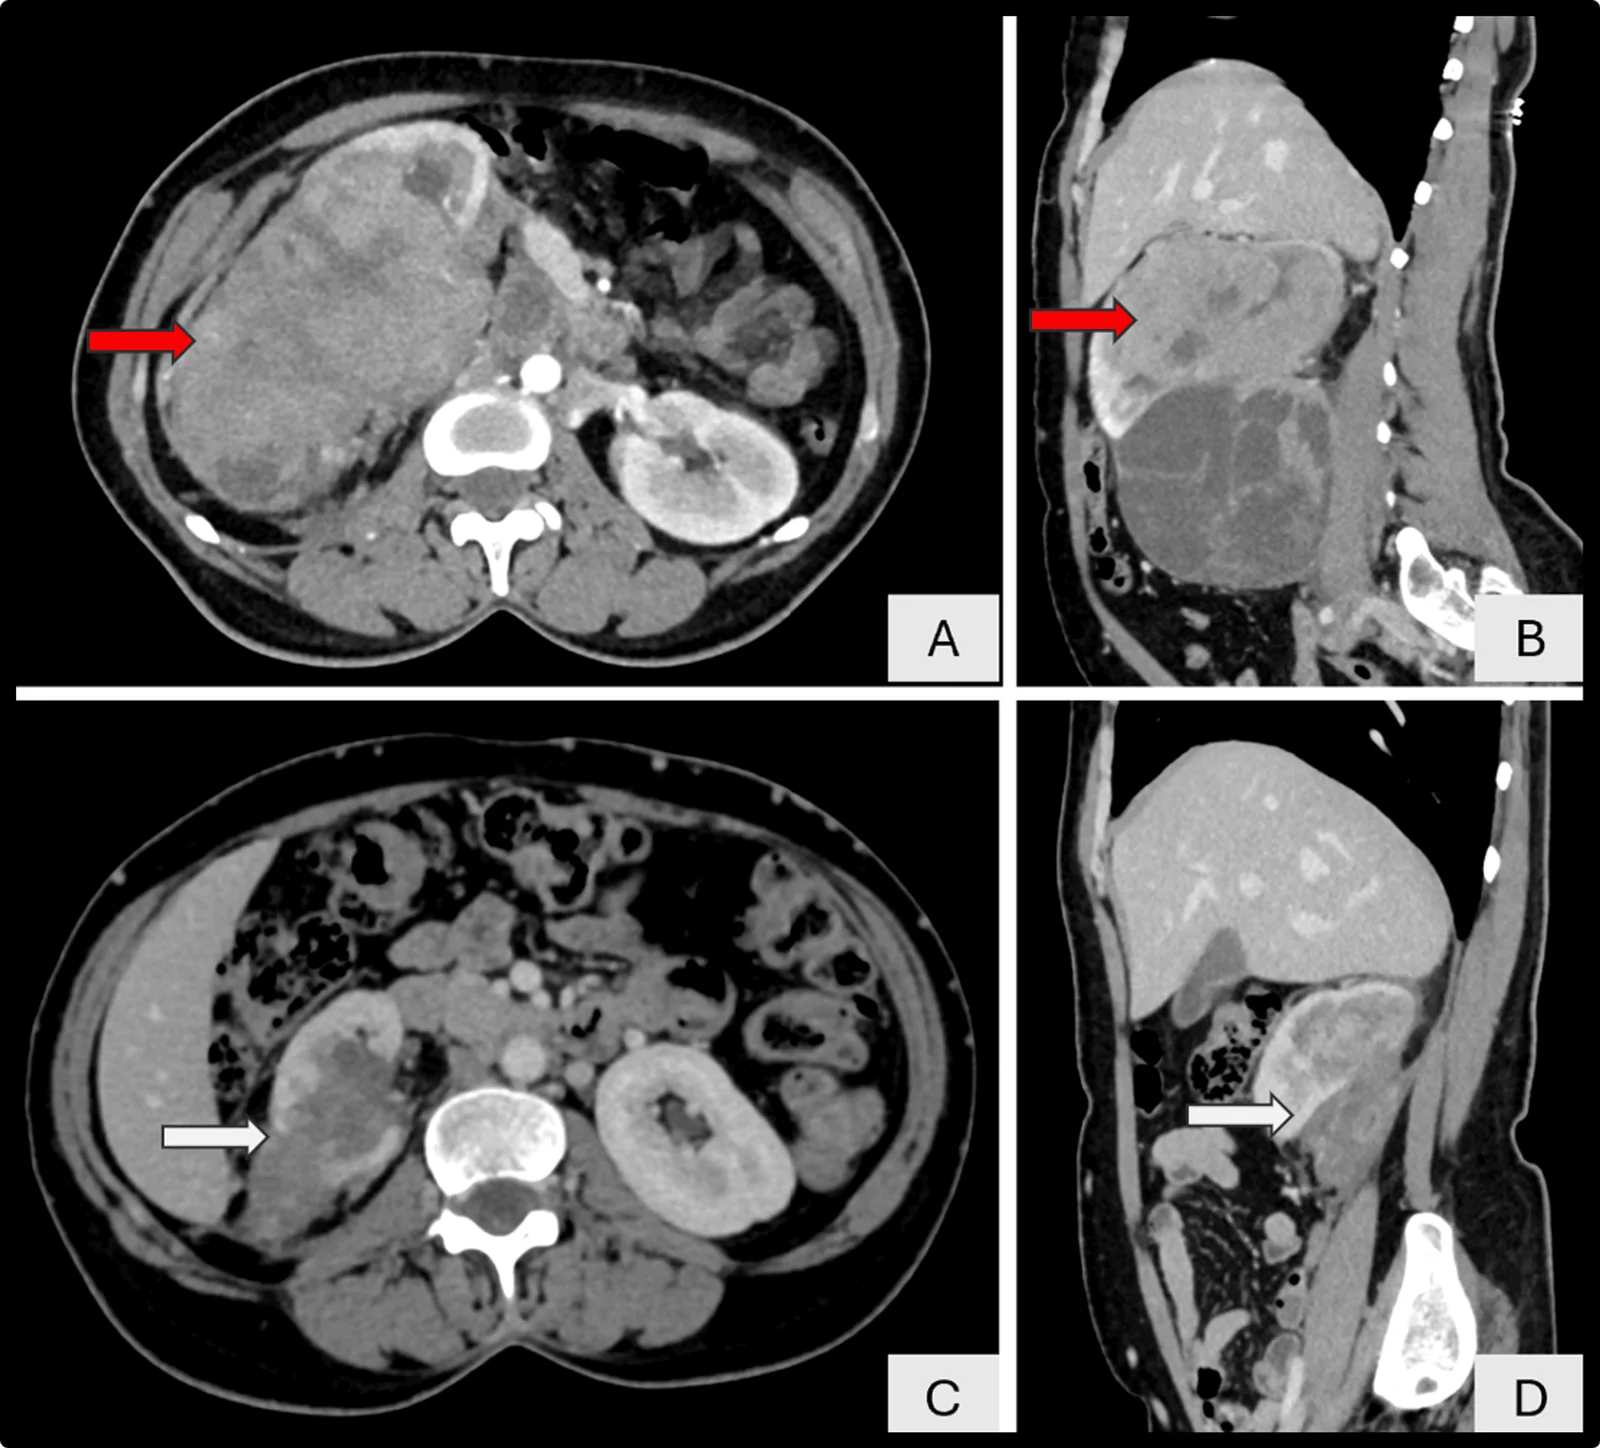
Pre (panels A and B) and post (panels C and D) chemotherapy changes A: Axial CECT image demonstrates a relatively well-defined heterogeneously enhancing right renal mass (red arrow) centered in the interpolar region, involving the corticomedullary region and adjacent calyces. The dilated IVC contains an enhancing tumor thrombus. B: Sagittal reconstructed image demonstrates the enhancing endophytic component (red arrow) and a predominantly necrotic exophytic posteroinferior component. C: Axial CECT image demonstrates a marked reduction in tumor size (white arrow) with partial resolution of the IVC tumor thrombus and reduction in IVC caliber. D: Sagittal reconstructed image demonstrates a significant reduction in the size (white arrow) of the predominantly necrotic exophytic component. CECT: Contrast-enhanced computed tomography, IVC: Inferior vena cava
